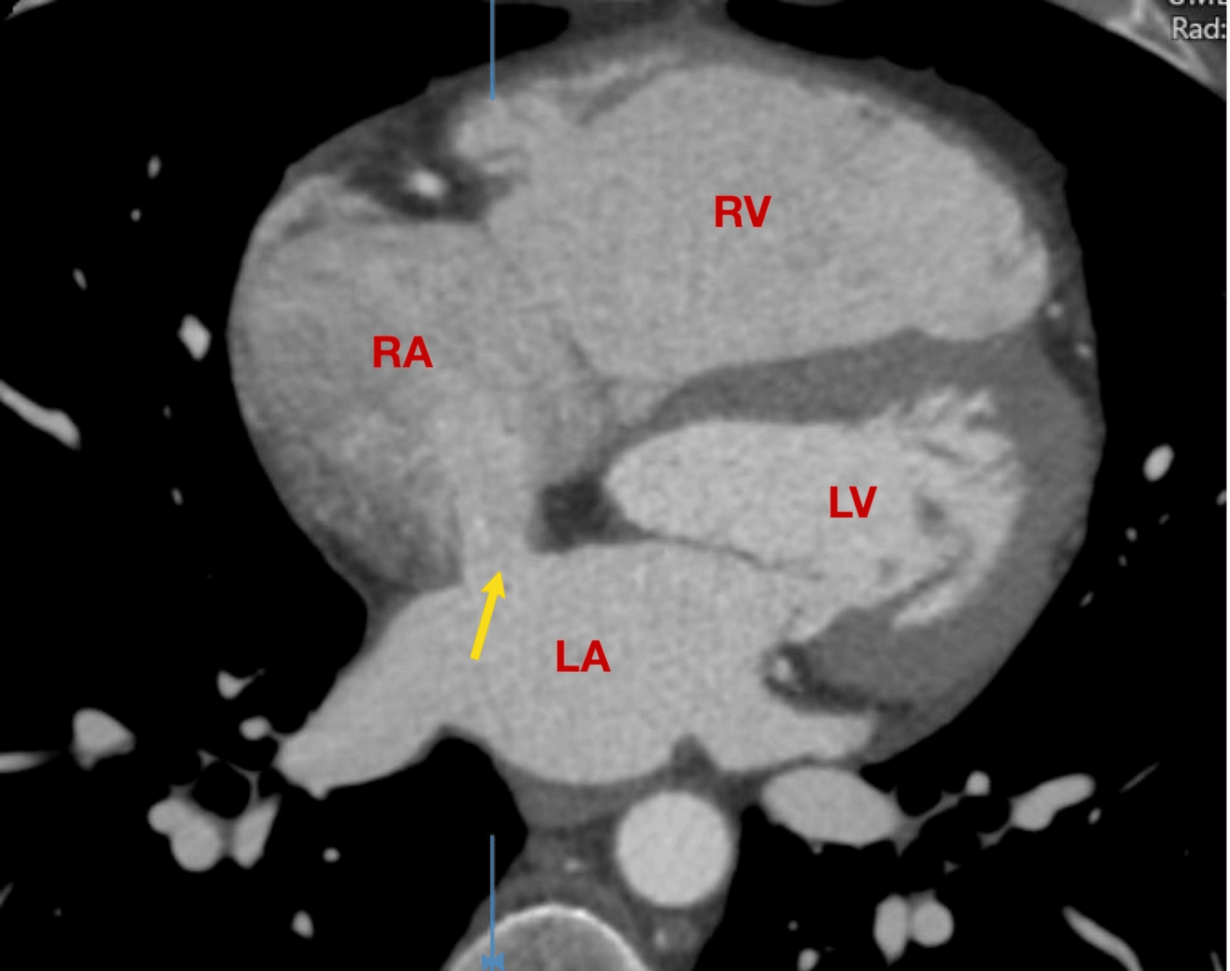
MDCT: four-chamber view demonstrating the atrial septal defect with a jet from the left to the right atrium. MDCT: multidetector CT; RV: right ventricle; RA: right atrium; LV: left ventricle; LA: left atrium
